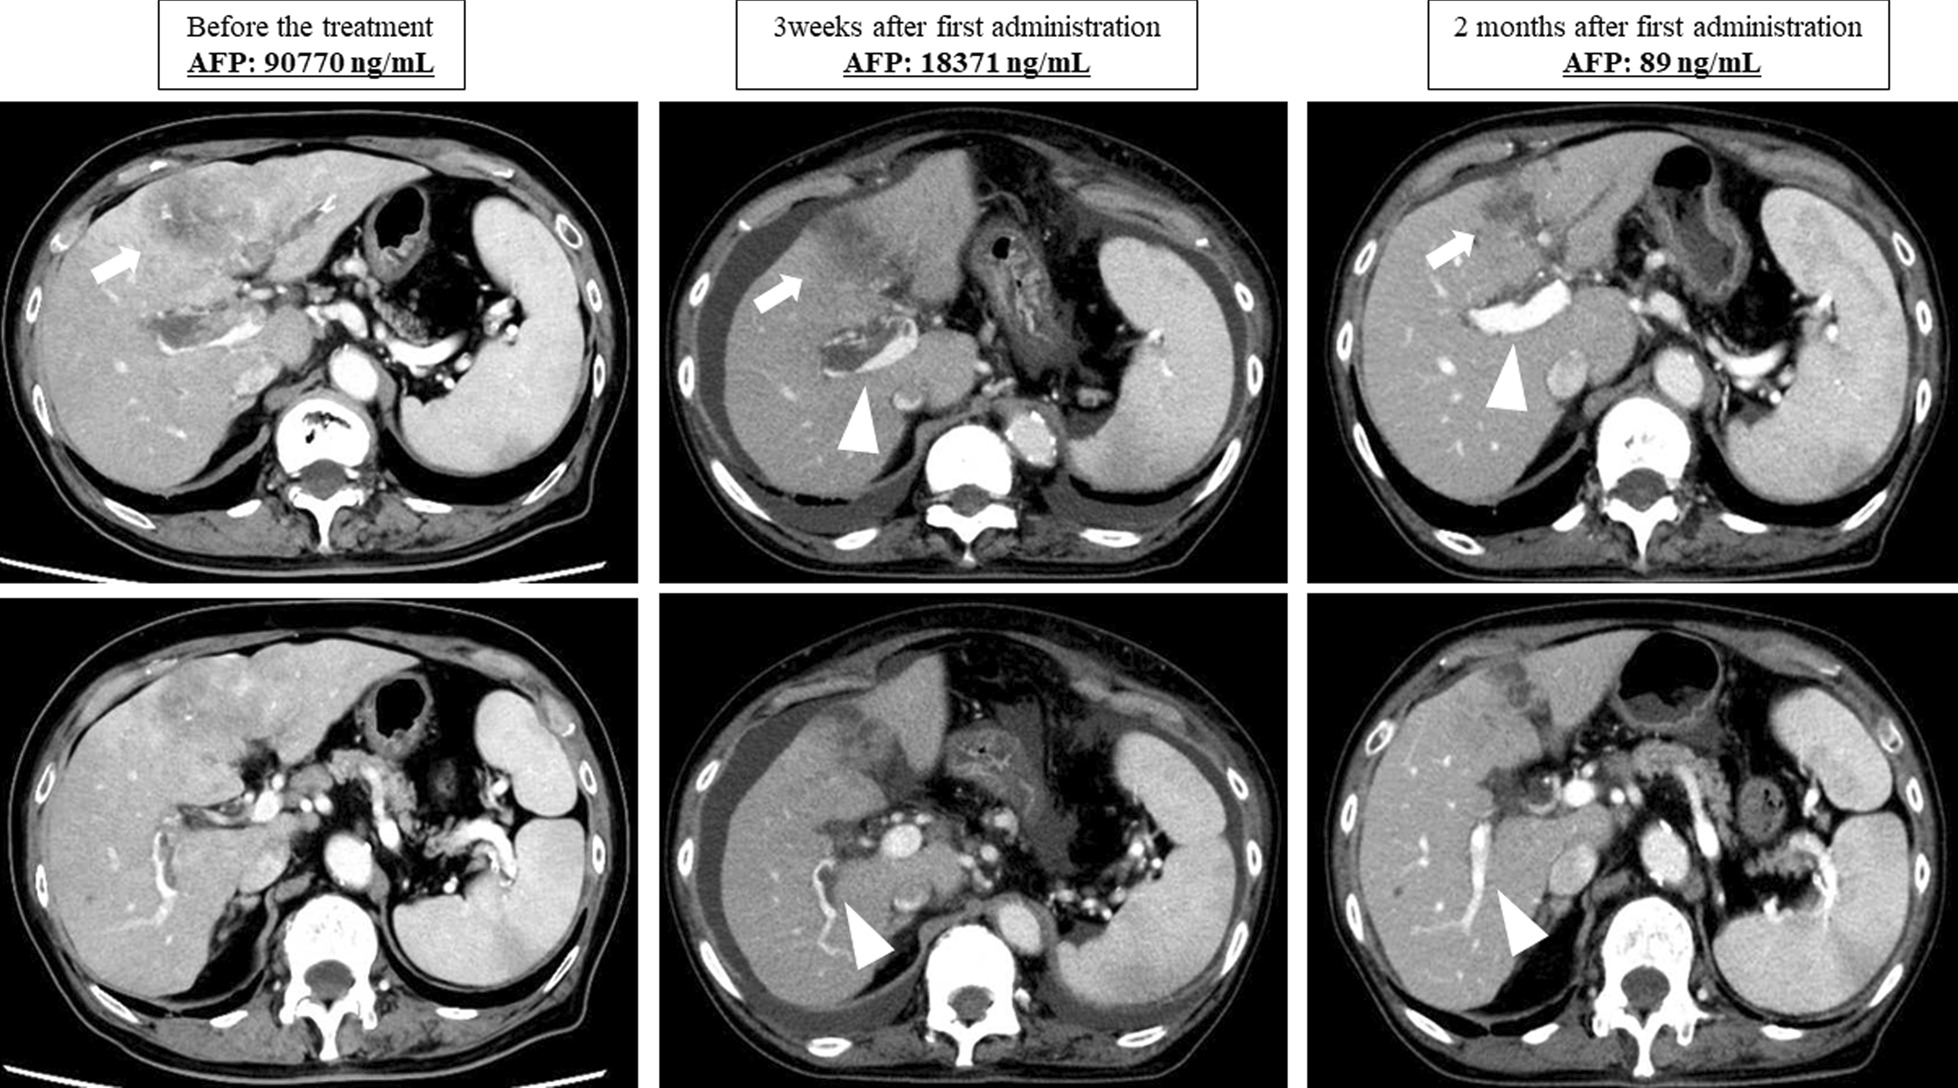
Abdominal computed tomography showing size reduction of the main tumor and the portal vein tumor thrombus (white arrow). The tip of the portal vein tumor thrombus has regressed from the bifurcation of the anterior and posterior branches to the left trunk, and the right portal vein is recanalized. Serum alfa-fetoprotein level decreased from 90,700 ng/mL before the treatment to 18,371 ng/mL (3 weeks later) and 89 ng/mL (2 months later). AFP, alfa-fetoprotein
